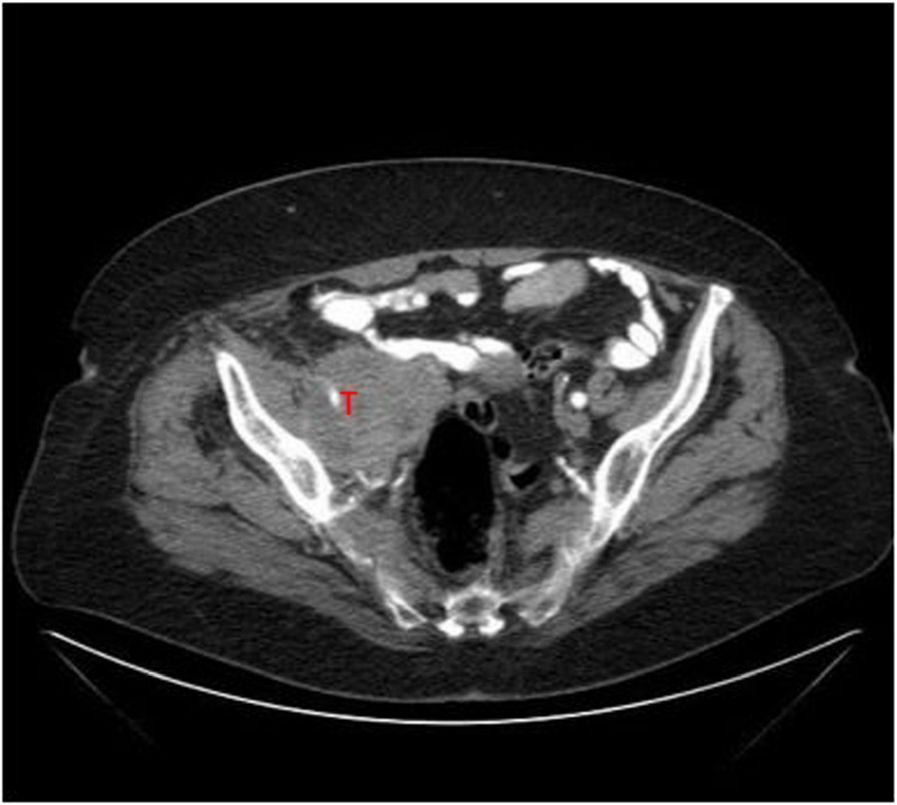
Transverse abdominal computed tomographic scan. *T* Tumor
